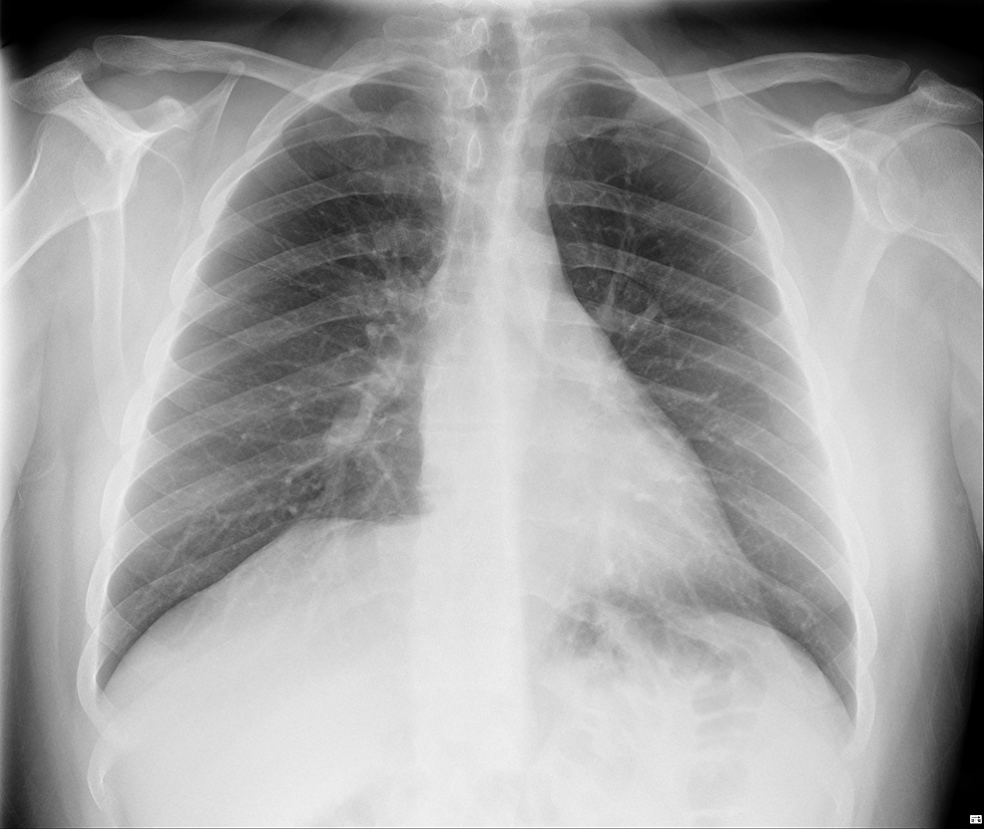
Normal chest X-ray of the patient
